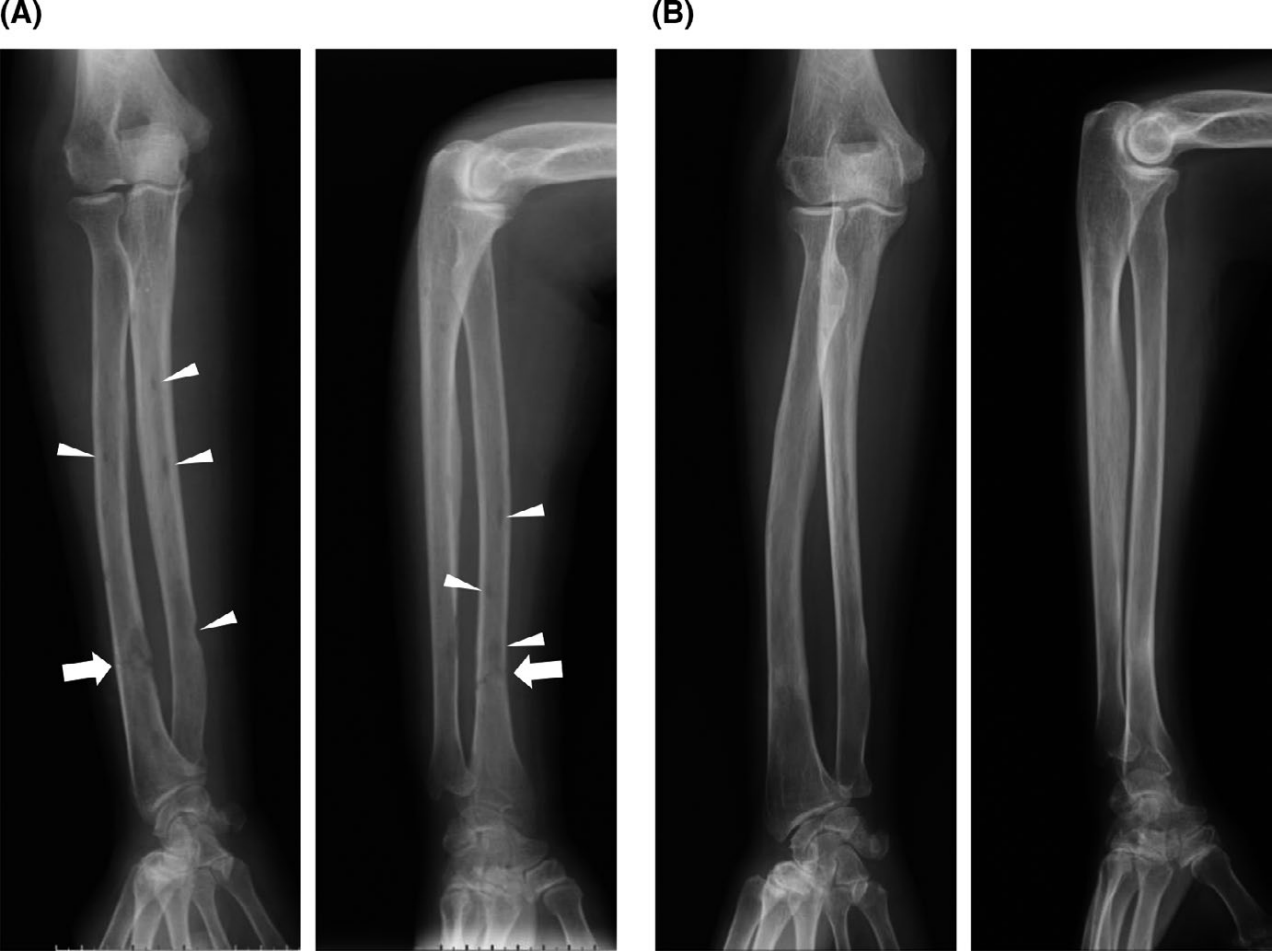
Plain radiographs of right forearm bones obtained before the initiation of chemotherapy (A) and 2 y after haplo‐HSCT (B). Arrows indicate the site of fracture of the radius. Osteolytic lesions are indicated by arrowheads
